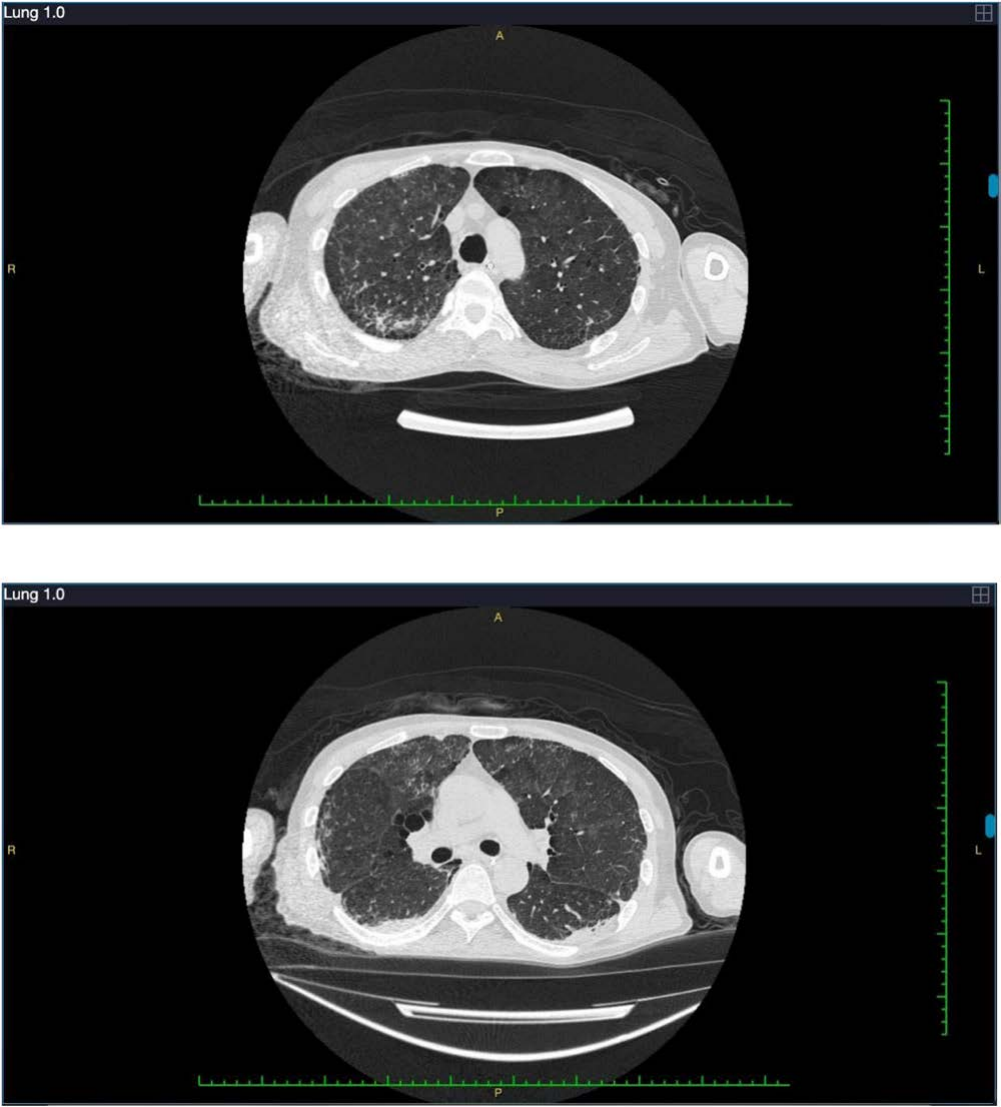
The chest computed tomography on the 29th day of hospitalization showed increased lung density, indicating a smaller lesion area than the 1 observed on the 22nd day of hospitalization.
